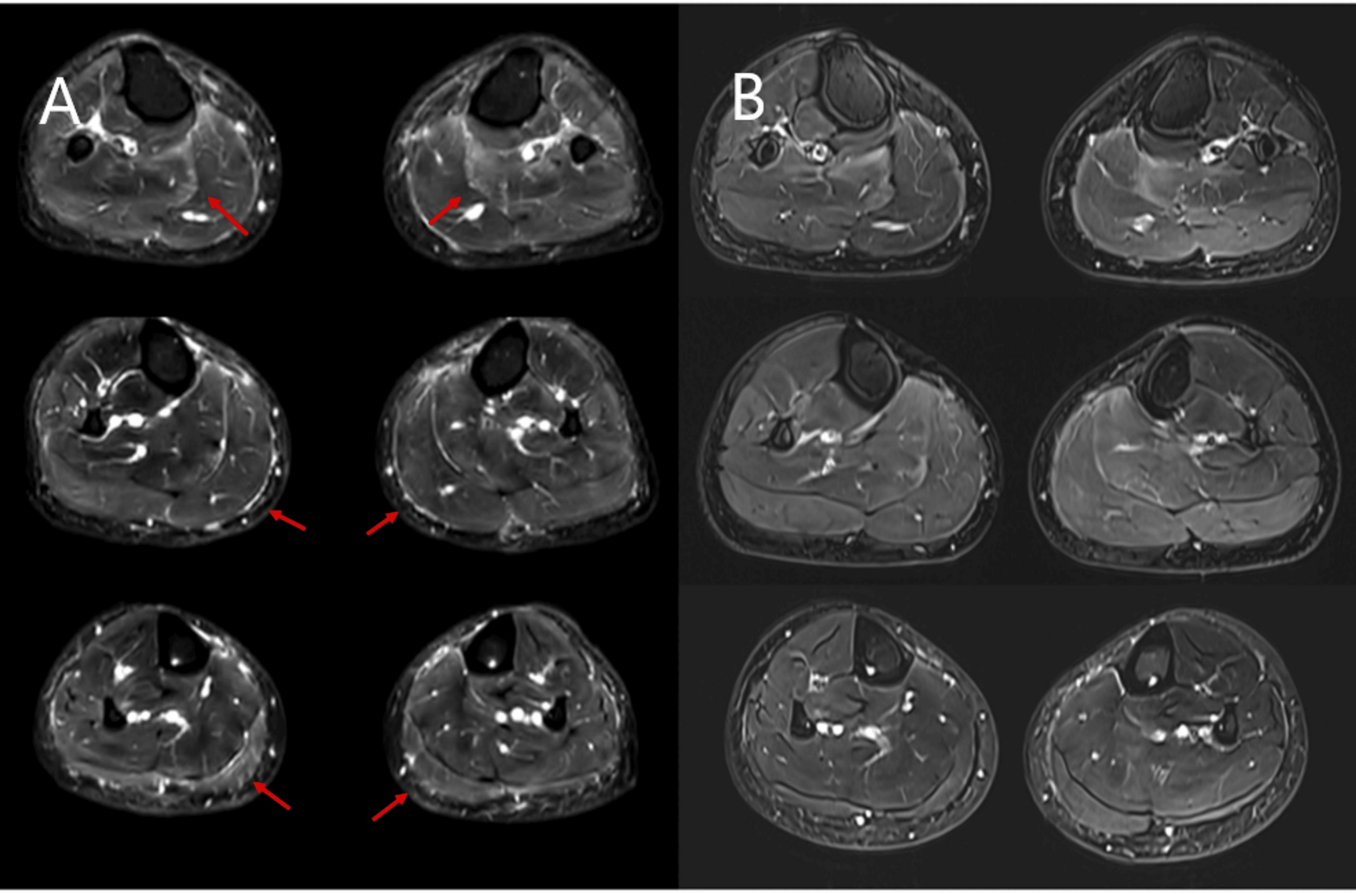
Bilateral lower legs on MRI before and after treatment Short Tau Inversion Recovery (STIR) images of the bilateral lower legs on MRI demonstrate high signal intensity in both gastrocnemius muscles before treatment, with particularly pronounced signals along the fascia (arrows). After treatment, these high-signal areas in the gastrocnemius muscles and fascia resolved. (A) Before treatment; (B) after treatment.
